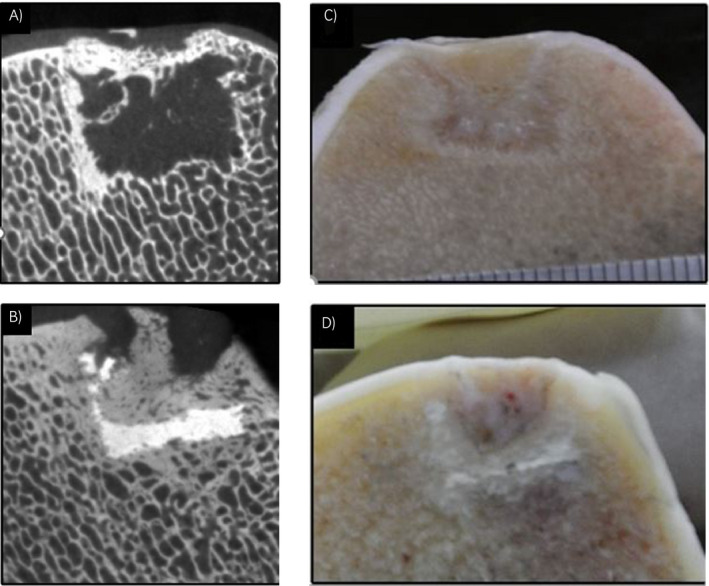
Micro‐CT and macroscopic pictures, respectively, of implantation sites at 6 mo. A) and C) created osteochondral defect treated with cartilage derived matrix (CDM) scaffold only: the void below the scaffold implant shows lacking bone repair. B) and D) created osteochondral defect treated with a combined CDM and Calcium phosphate scaffold: good integration of the scaffold within the bony portion of the defect^3^
